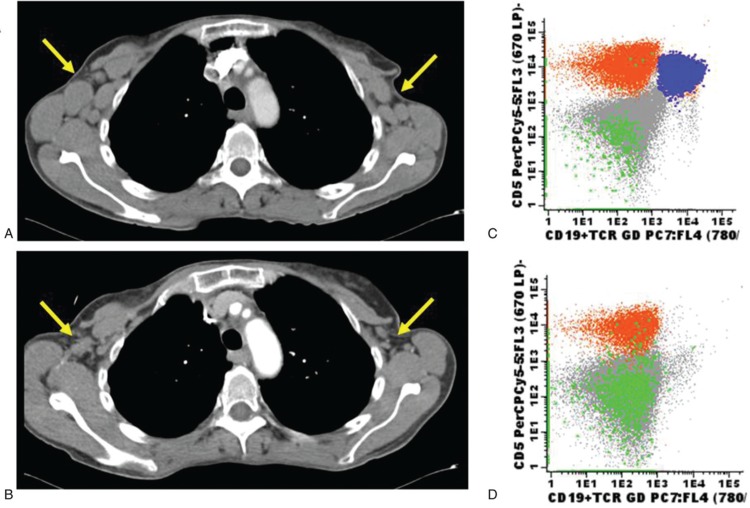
Panels A and B show a chest computed tomography from the patient before (A) and after (B) ARI-0001 infusion. Yellow arrows point to bilateral axillary lymphadenopathy remarkably improving upon therapy. Panels C and D show flow cytometry plots from bone marrow aspirates before (C) and after (D) ARI-0001 infusion. CLL cells are depicted in blue while normal T and NK-cells are depicted in green and yellow, respectively. Notice that the patient had received the anti-CD20 monoclonal antibody obinutuzumab right before study inclusion, thus explaining the lack of normal (CD19^+^/CD5^−^) B cells even before ARI-0001 infusion. CLL = chronic lymphocytic leukemia.
